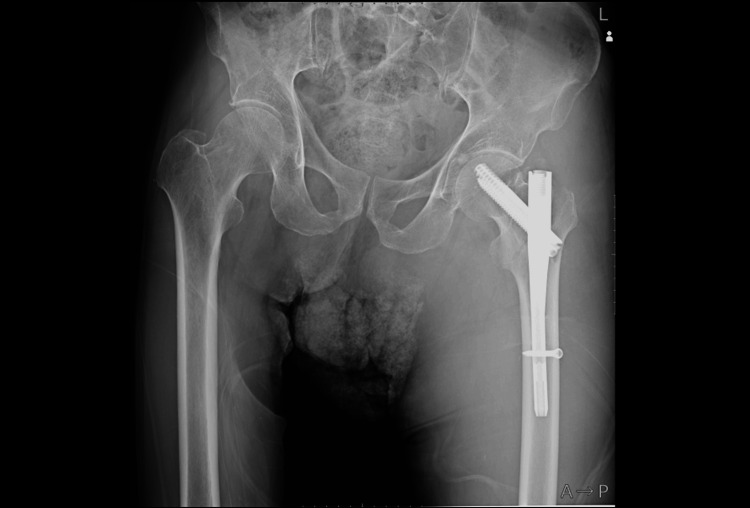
Plain radiograph on postoperative day 7 The affected thigh is markedly swollen
